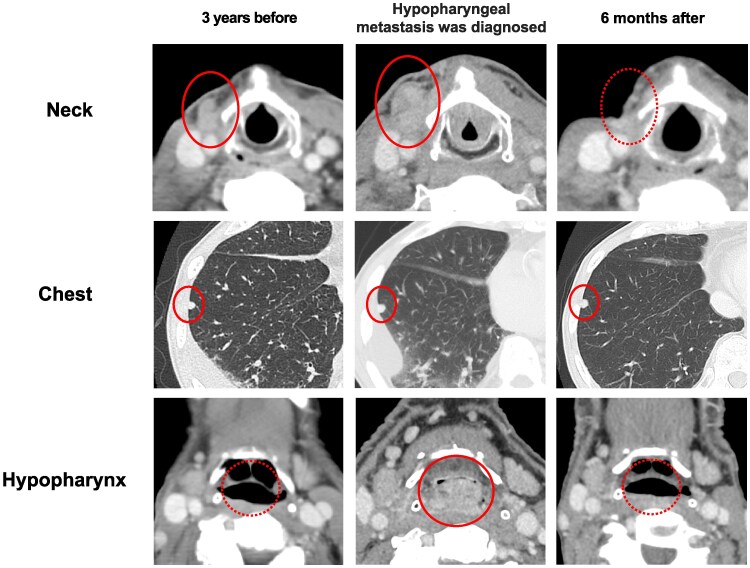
Serial CT imaging from 3 years before treatment to 6 months after treatment. Serial computed tomography (CT) images showing disease progression and treatment response. Upper row: Contrast-enhanced cervical CT images at 3 years before, at current presentation, and 6 months after, showing changes in the anterior cervical lymph node metastasis. Middle row: Chest CT images at 3 years before, at current presentation, and 6 months after, showing lung metastasis. Lower row: Contrast-enhanced hypopharyngeal CT images at 3 years before, at current presentation, and 6 months after, showing changes in the hypopharynx metastasis.
